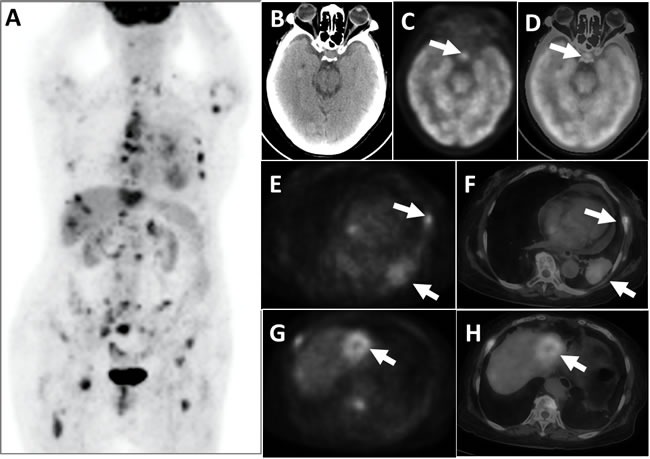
This patient suffered from lung cancer, combined with multiple systemic metastases, had pituitary hypermetabolism PET whole-body images (MIP) appeared multiple hypermetabolic A. PET cross-sectional imaging and CT fusion images revealed space-occupying lesions on the left lower lobe of the lung combined with adjacent rib bone destruction and hypermetabolism E. and F., white arrows); a space-occupying hypermetabolism was visible on the left liver lobe G. and H., and liver metastasis was suspected (white arrows). Brain PET C., CT B., and the fusion of cross-sectional tomographic images D. revealed that the pituitary exhibited FDG hypermetabolism (white arrows), with an SUVmax of 7.3.
